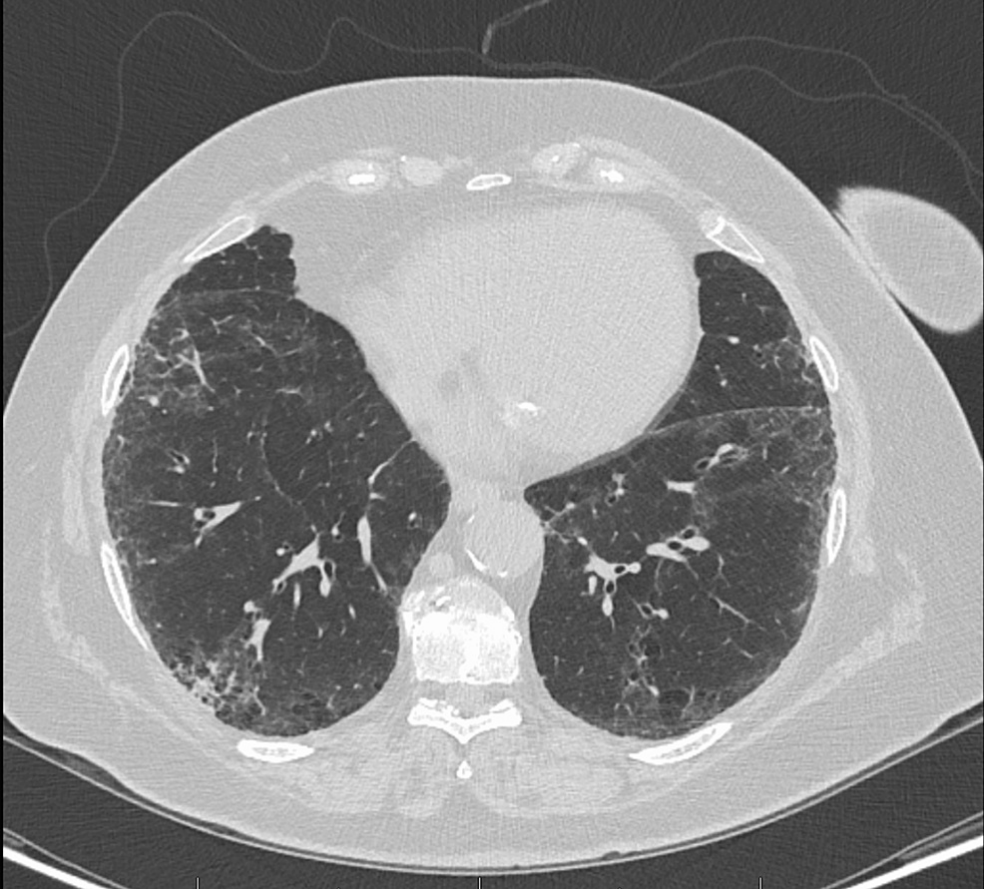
Chest CT showing evidence of lung fibrosis with subpleural fibrotic changes, peripheral honeycombing, and small areas of traction bronchiectasis
